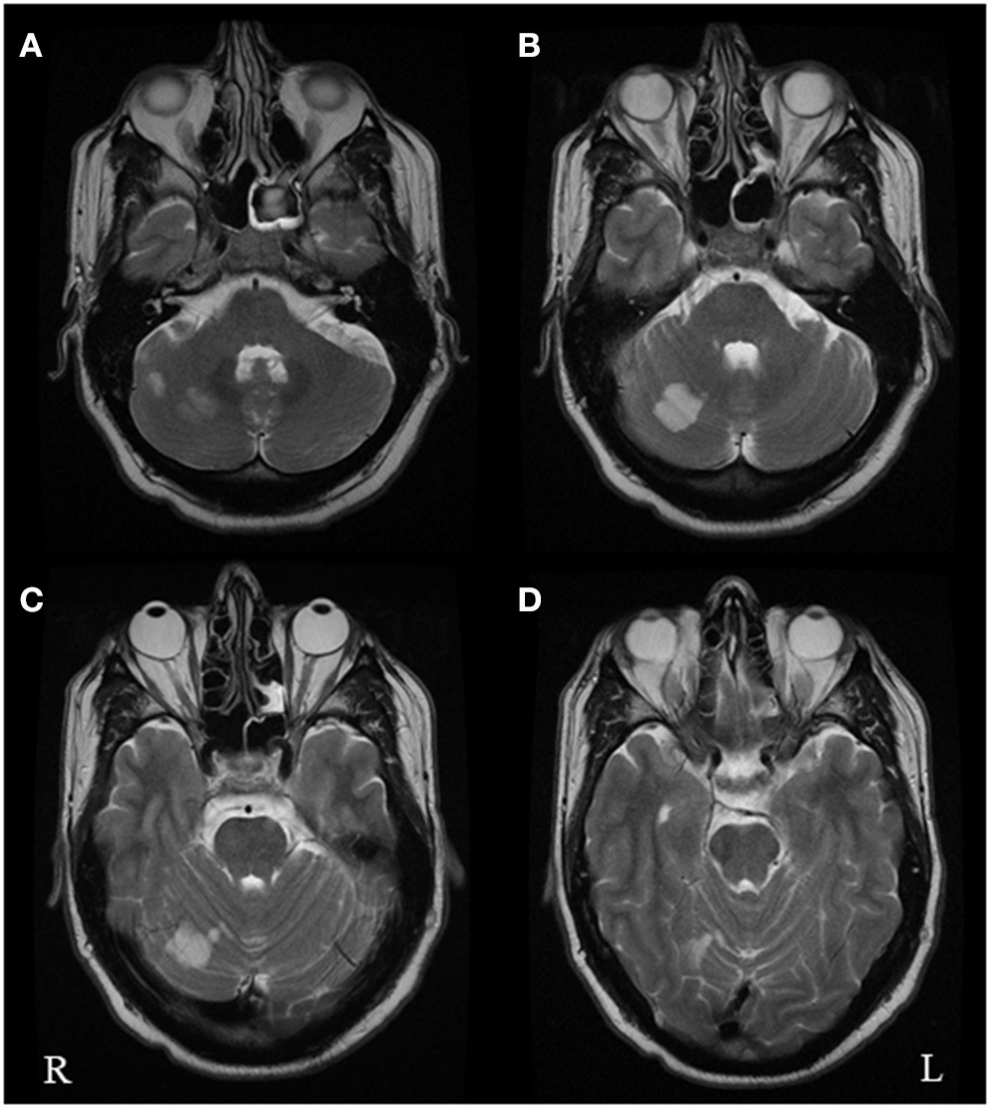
Axial T2 brain MRI images performed in October 2019 following the acute vascular event. The images show multiple foci of restricted diffusion in the right superior cerebellar hemisphere (A–C) within the territory of the right superior cerebellar artery, consistent with acute infarcts. There was no restricted diffusion within the left occipital lobe (D).
